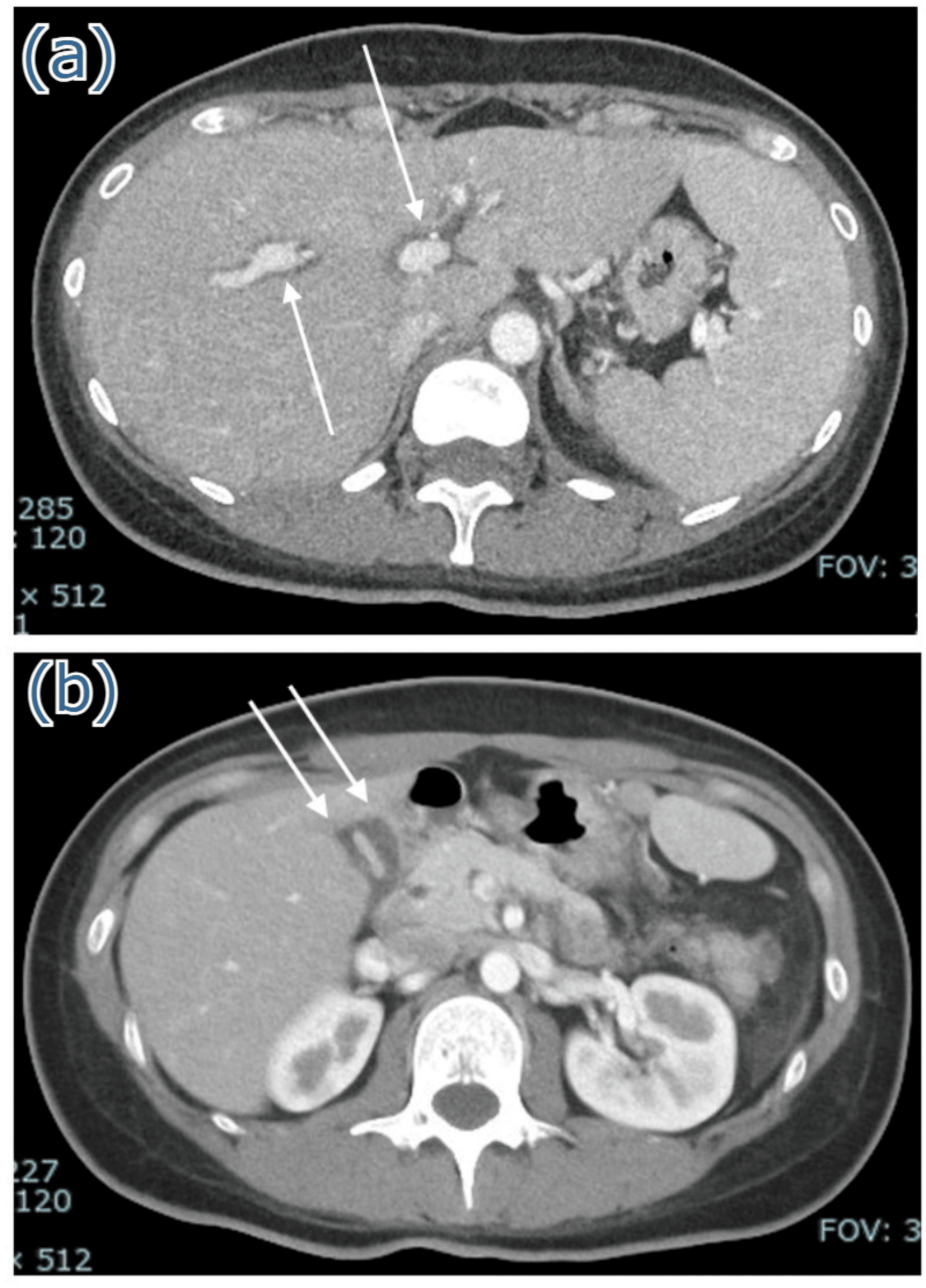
Computed tomography images. Abdominal contrast-enhanced computed tomography showing (a) hepatosplenomegaly and periportal collar sign in the liver (white arrows), and (b) subserosal edema of the gallbladder (white arrows).
